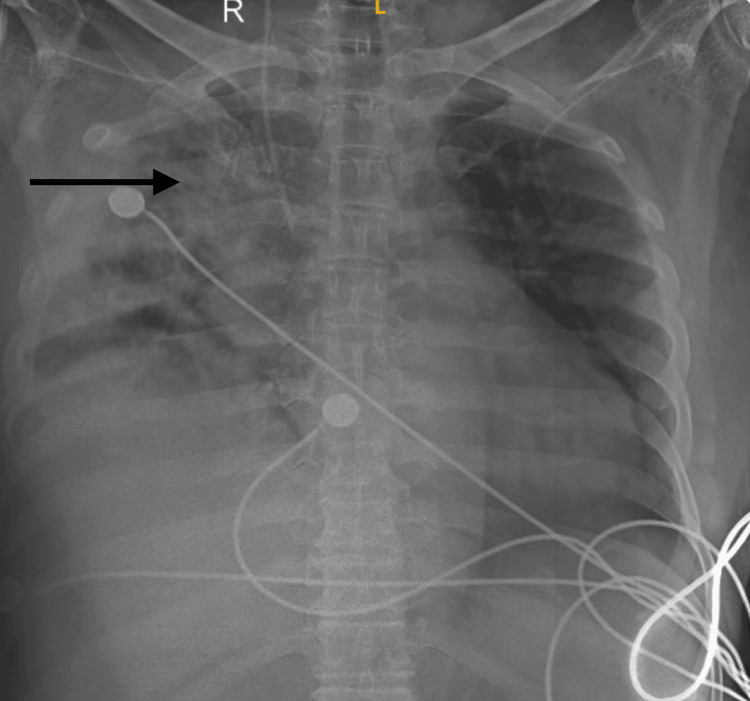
Chest X-ray showing opacities in the right upper lobe with surrounding consolidation.
